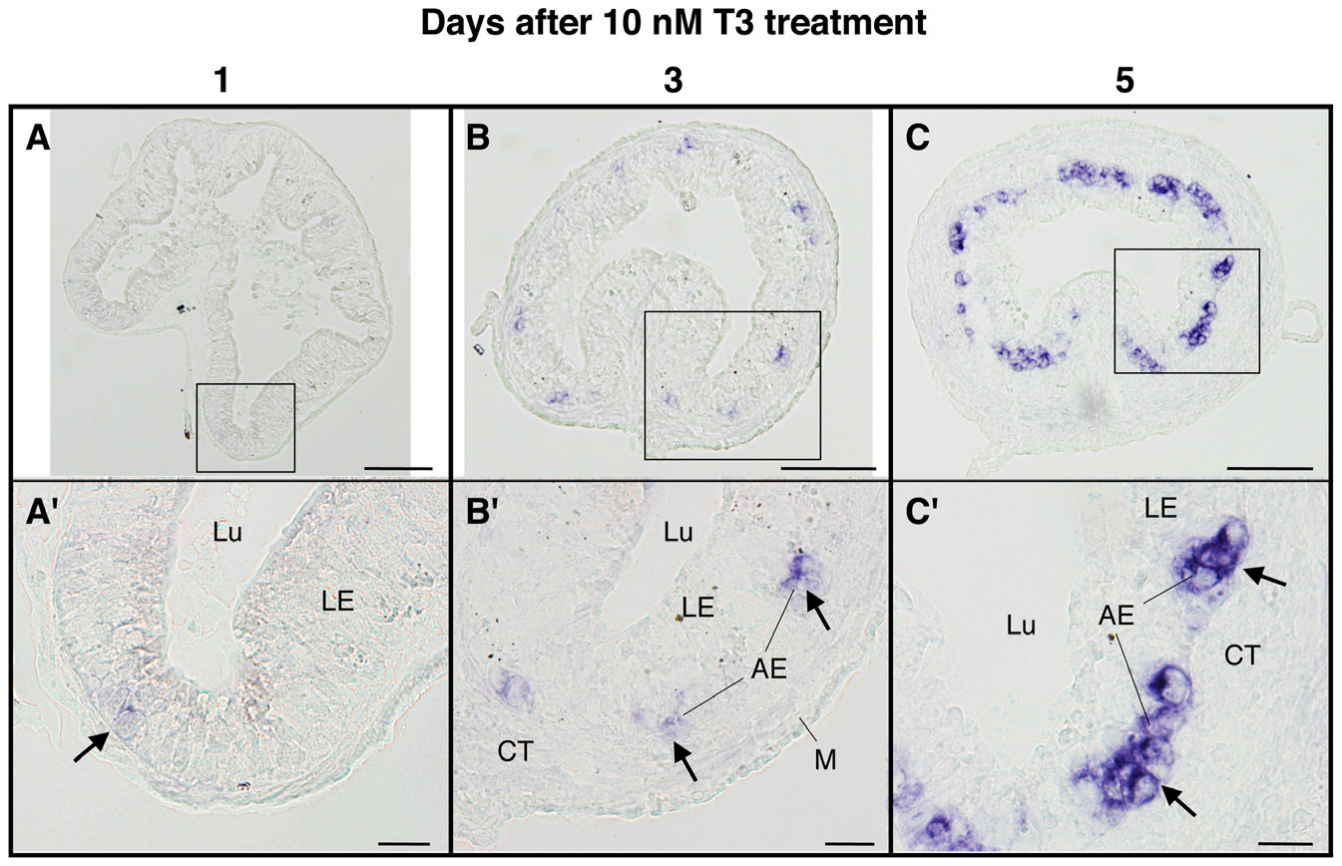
T3-dependent expression of LGR5 mRNA in the small intestine. Cross sections of the intestine from premetamorphic tadpoles (stage 54) treated with 10 nM T3 for 1 (A, A′), 3 (B, B′) and 5 days (C, C′) were hybridized with LGR5 antisense probe. AE: adult epithelial cell including the progenitor/stem cell, CT: connective tissue, LE: larval epithelial cell, Lu: lumen, M: muscle layer. Scale bars are 100 µm (A–C) and 20 µm (A′–C′), respectively.
